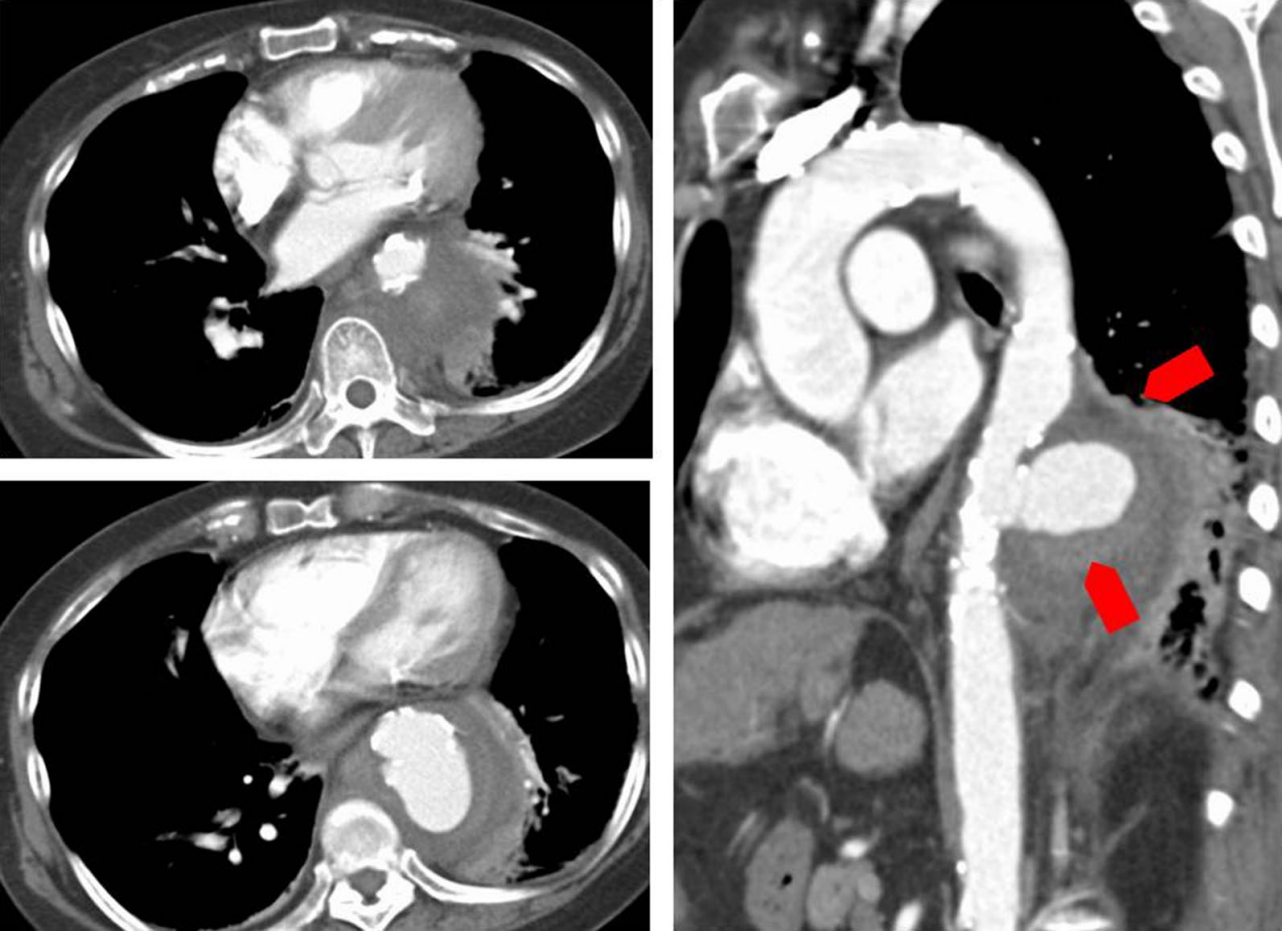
Contrast enhanced CT scan of the descending aorta on admission. There is a ruptured aortic aneurysm of the descending aorta
